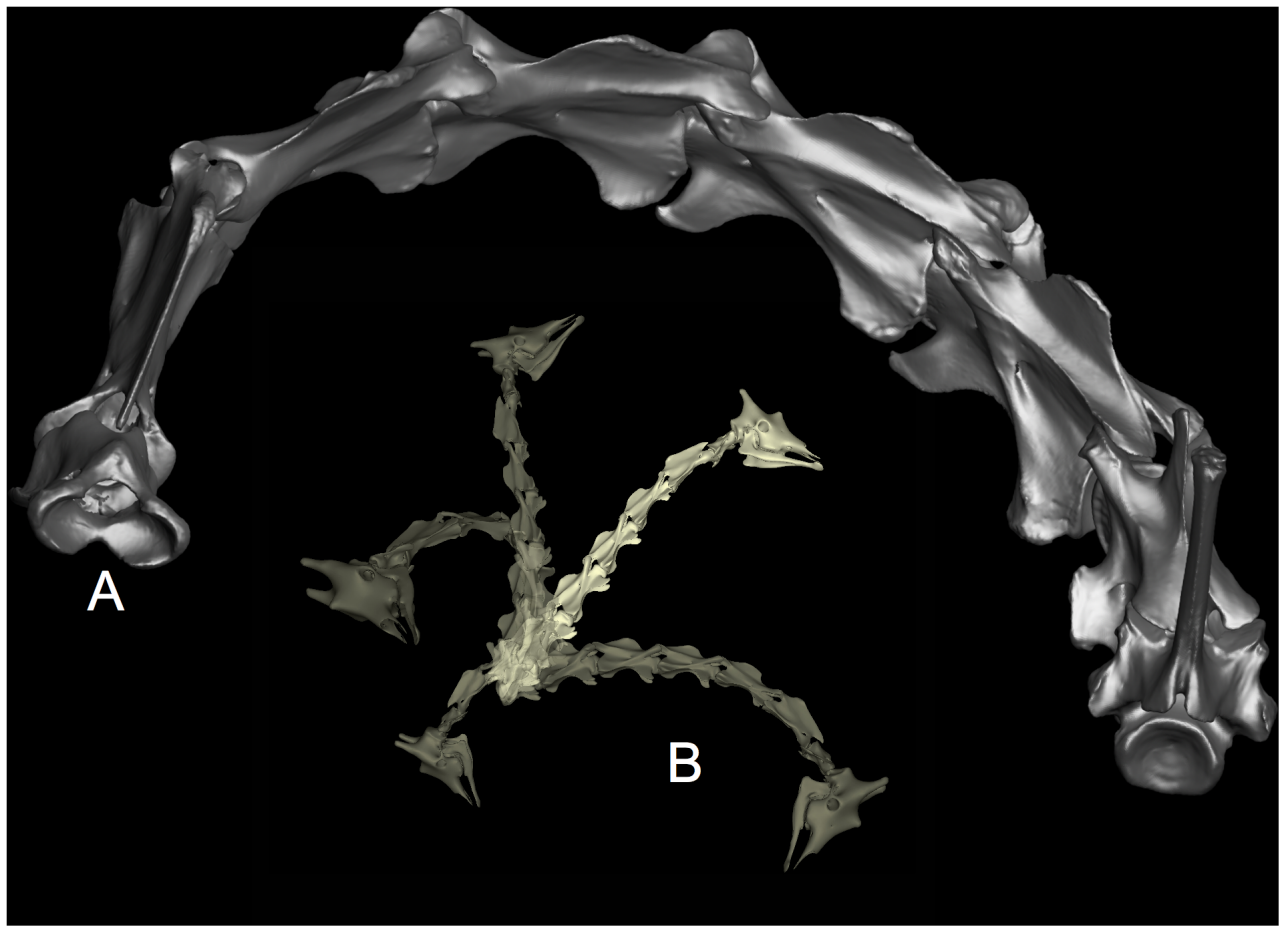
Giraffe flexibility is predicted by their joint geometry. The ability of a giraffe to reach vertically and to flex laterally to just reach its flanks is closely replicated by a digital model based on CT scan data of a recent giraffe (see also closeup in Figure 11). The zygapophyses remain in articulation with substantial overlap when they reach osteological stops at the base). CT data provided courtesy American Museum of Natural History and Timothy Rowe, University of Texas. Supplemental material: Movie S4.
